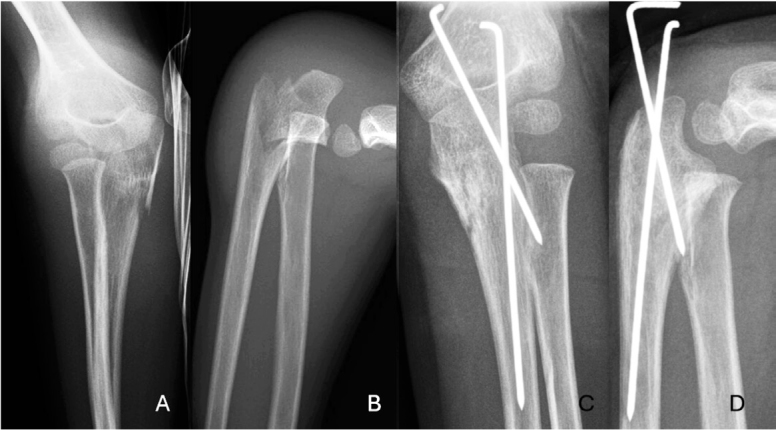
A 5-year-old boy, with left hand injury. In the radiographs a proximal ulnar fracture and posterior luxation of the radiohumeral joint was registered (A, B). This was classified as Bado type II Monteggia fracture. Injury was treated with Kirschner wires, which were afterwards removed (C, D). Injury had healed fully 7 years later when the patient was interviewed via phone.
